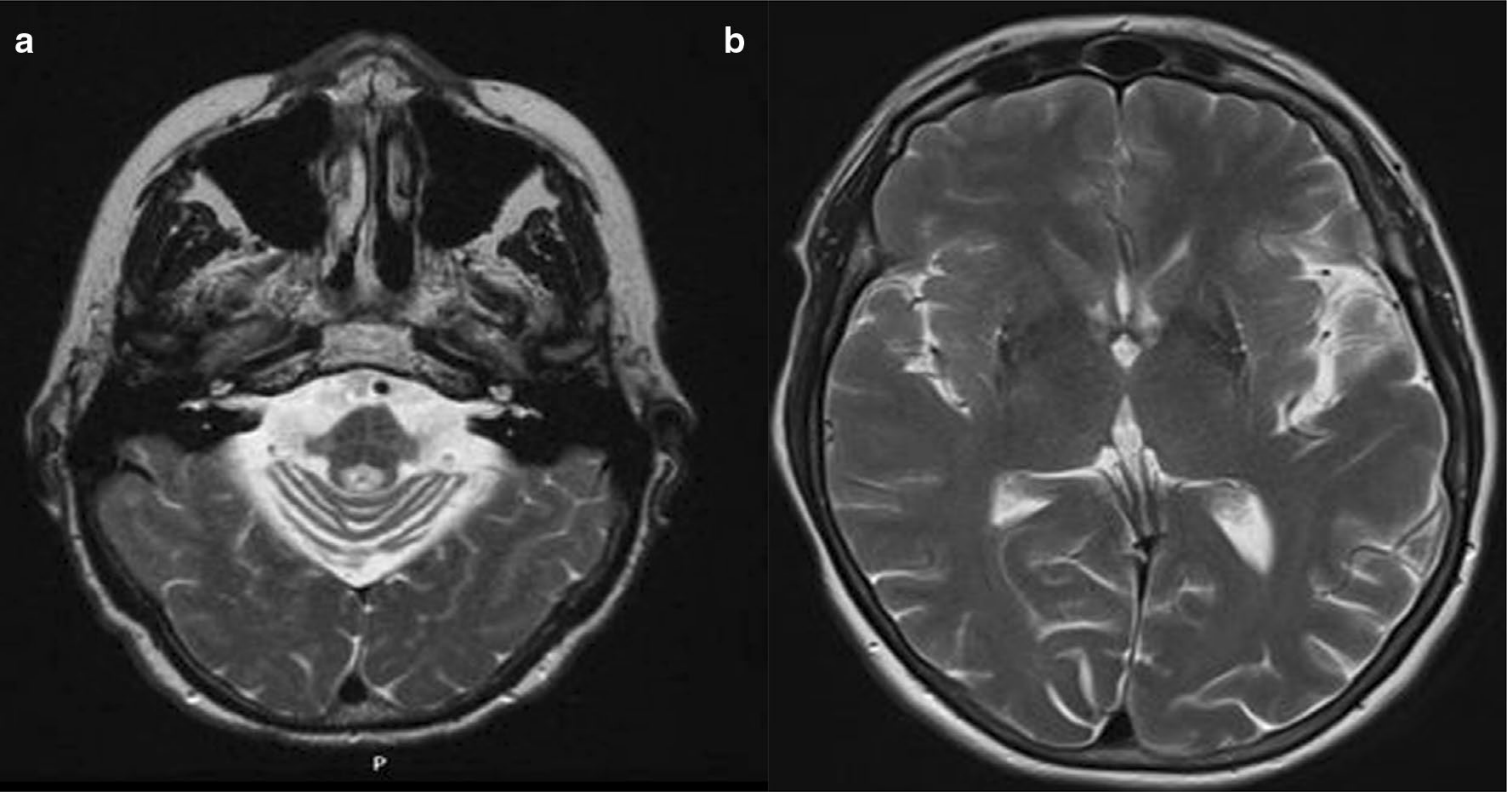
a ‘‘Hot cross-bun sign’’ seen on an axial T2-weighted MRI in a patient with MSA-C; b putaminal hypointensity with a hyperintense “putaminal rim” sign on an axial T2-weighted MRI in a patient with MSA-P. Images adapted from [9]
